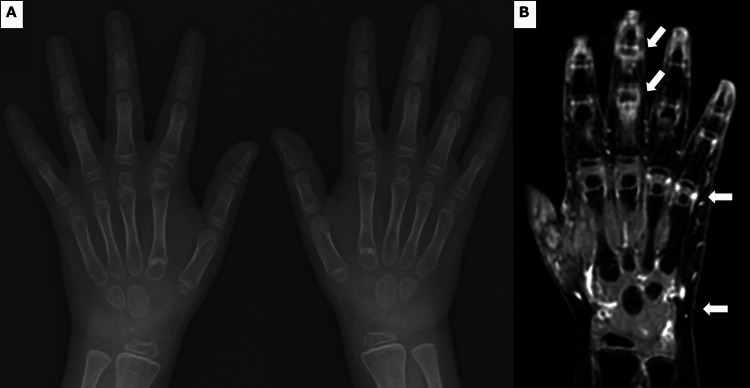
Imaging findings of the hands and wrists at the onset of juvenile idiopathic arthritis (A) Anteroposterior radiograph of both hands showing no evident bone erosions or joint space narrowing. (B) Contrast-enhanced T1-weighted magnetic resonance imaging of the wrists and fingers revealing active synovitis and joint effusion. White arrows indicate active synovitis and joint effusion.
